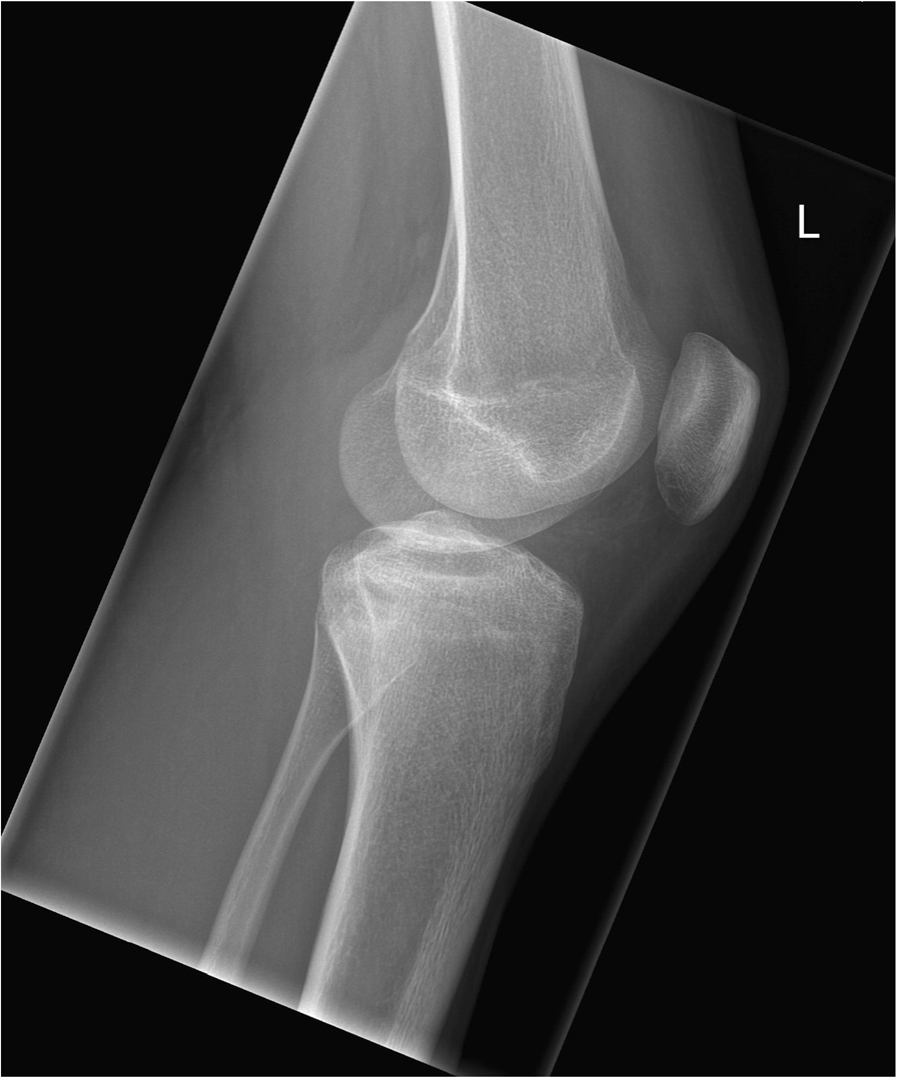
X-ray of the left knee (lateral view), taken at the first postoperative out-patient presentation (10 weeks postoperative)
